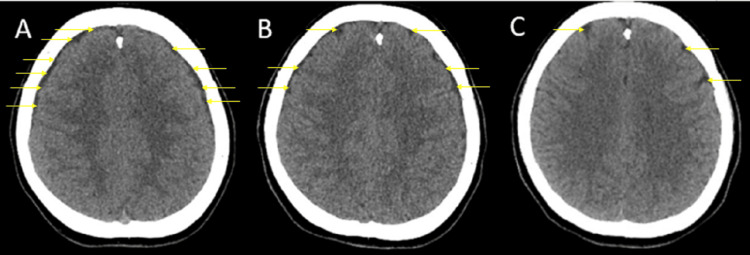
Computed tomography images A) Bilateral subdural hematoma on the fifth postoperative day; B) Bilateral subdural hematoma had regressed slightly on the sixth postoperative day; C) Almost completely resolved subdural hematoma on the eighth postoperative day The arrows indicate the bleeding areas. Serial imaging demonstrates a progressive reduction in the subdural hematoma.
